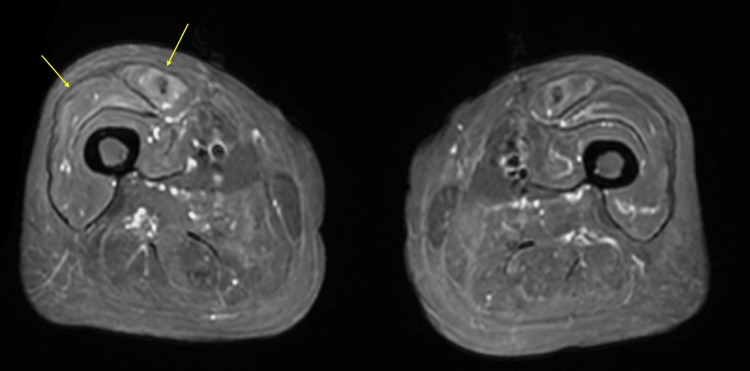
Short τ inversion recovery (STIR) sequence of muscle magnetic resonance imaging (MRI) of the thigh. The muscle MRI reveals hyperintensities in the right rectus femoris and vastus lateralis (arrows), which are consistent with immune-mediated necrotizing myopathy (IMNM).
